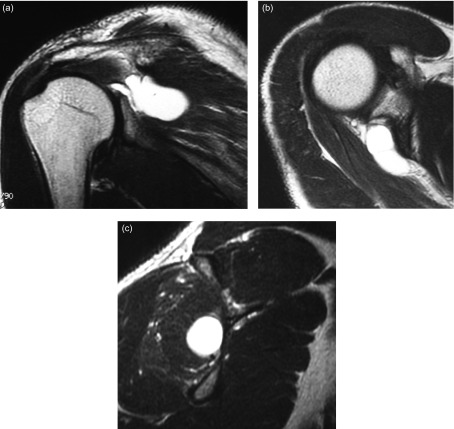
T2-weighted MRI scans of a left shoulder demonstrating a spinoglenoid cyst on the coronal image (a), the axial image (b), and the sagittal image (c). The sagittal image shows edema and atrophy of the infraspinatus muscle.
